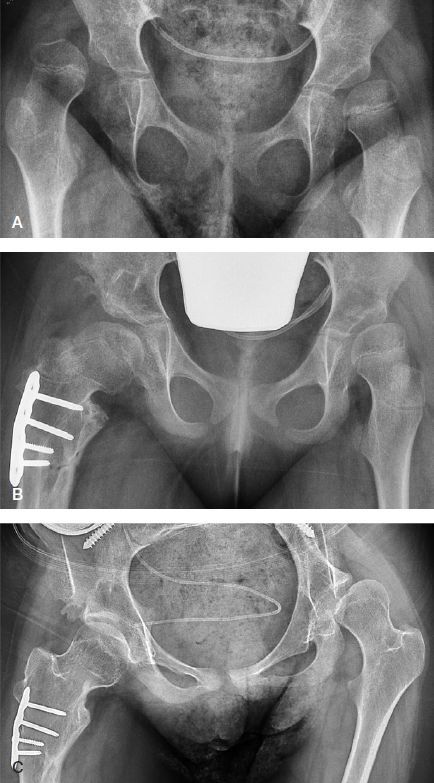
A. Preoperative radiograph of a girl, aged 5.7 years and GMFCS level V, with complete dislocation of her right hip (MP 100%) and subluxation of her left hip (MP 51%). B. 14 months after femoral and pelvic osteotomies of the right hip and bilateral soft tissue releases, showing slight subluxation of both hips (MP right hip 36% and left hip 37%). C. 7.3 years postoperatively (age 13.0 years), showing good position of right hip and deterioration of left hip (MP 64%).
